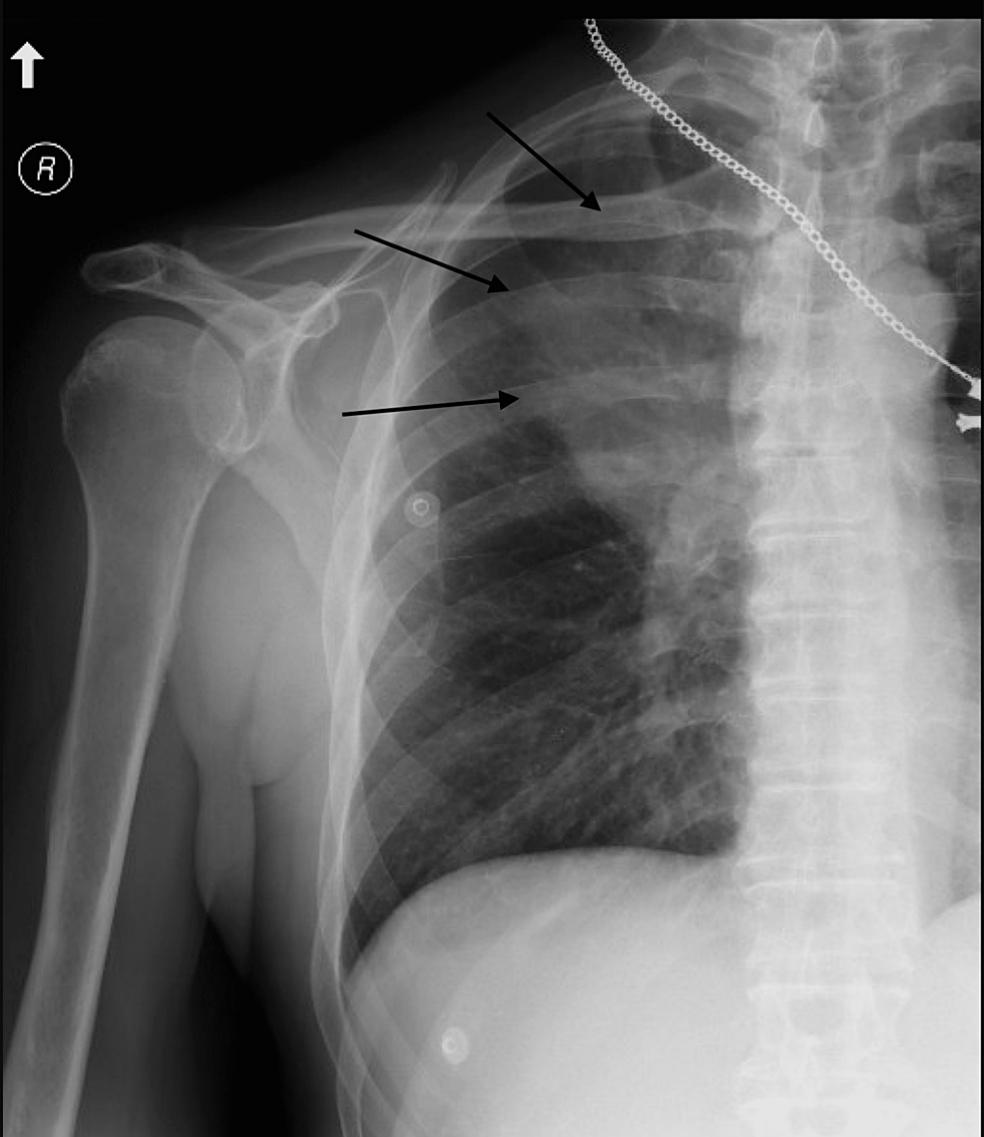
Second radiograph shows a well-defined opacity in the upper zone.
